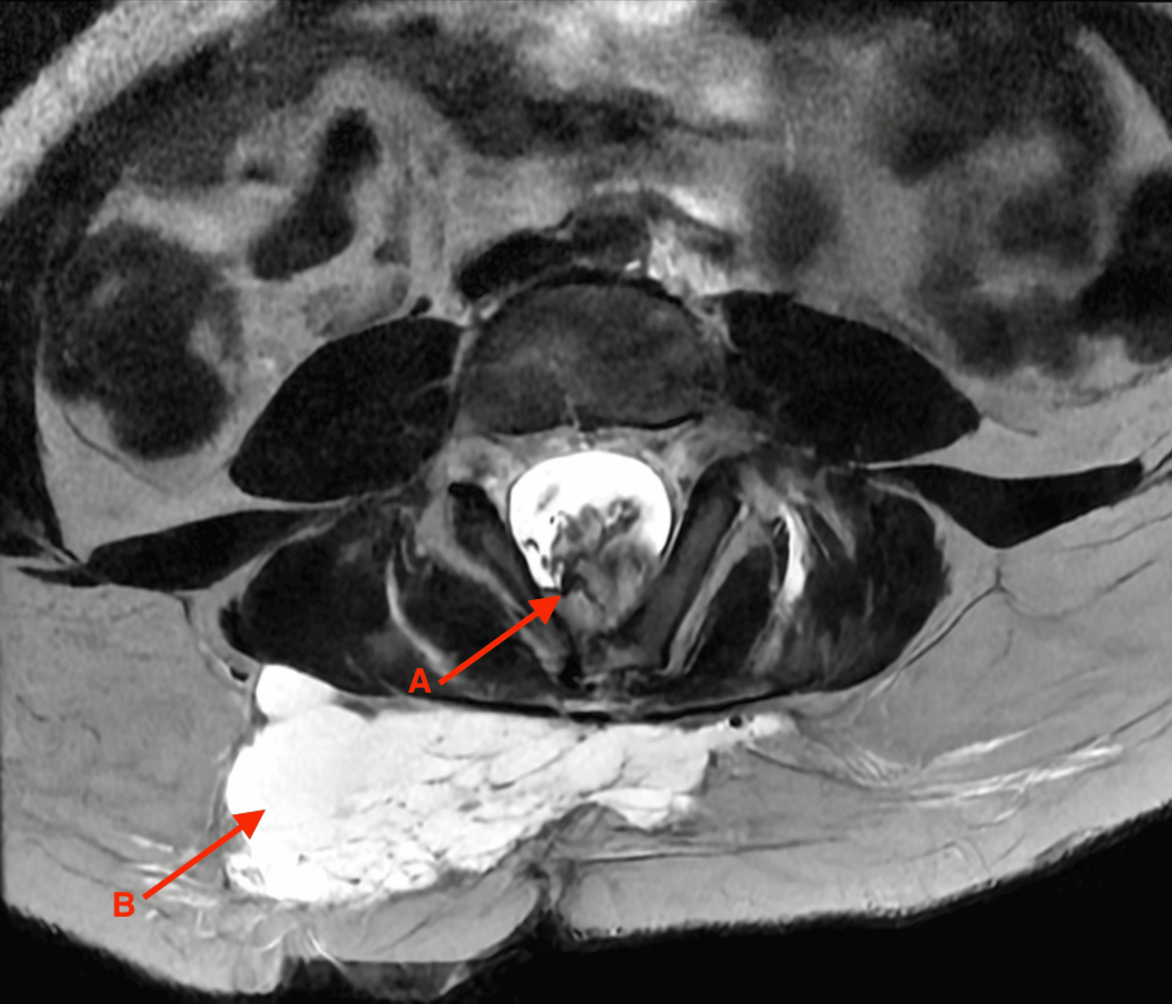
Axial lumbar magnetic resonance imaging A: Conus medullaris in continuity with hyperintense lipomatous tissue within the spinal canal. B: Hyperintense lipomatous tissue through a bony defect into the subcutaneous tissue, consistent with lipomyelomeningocele.
